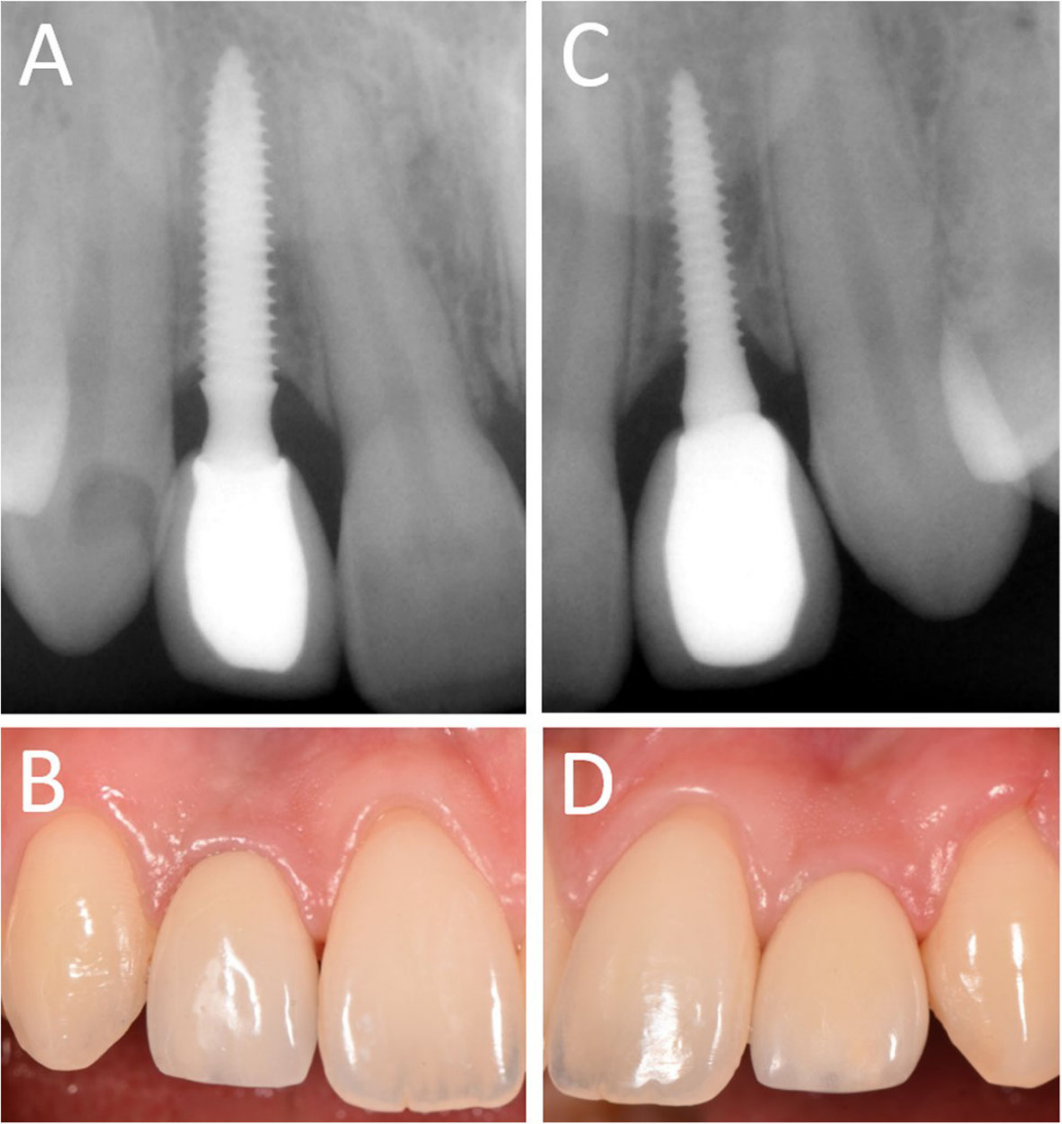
1-year follow-up control. a Right lateral incisor, radiographic control; b Right lateral incisor, frontal view. Note the soft tissues maturation; c Left lateral incisor, radiographic control; d Left lateral incisor, frontal view. Note the soft tissues stability
